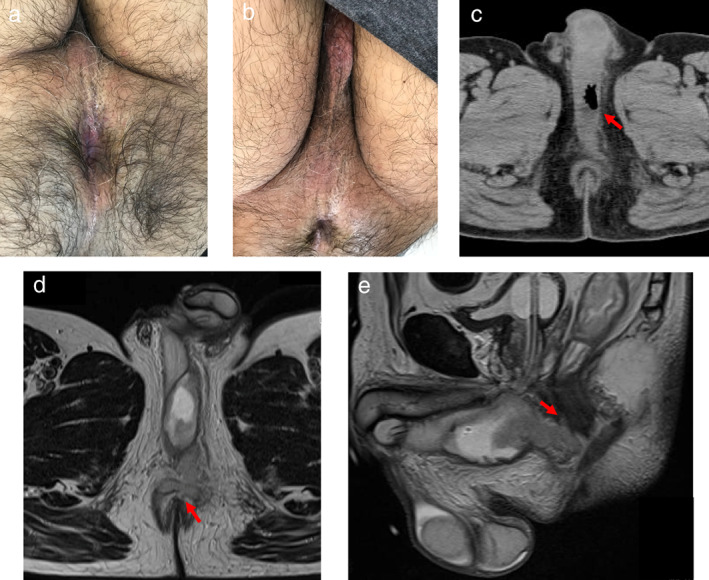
(a) No perianal redness is present, (b) but there is mild erythema of the perineum. (c) Computed tomography demonstrates a low‐density area with air in the perineum (red arrow). (d) Magnetic resonance imaging reveals continuity of the abscess with the anal canal at 12 o' clock (red arrow), (e) which is confirmed on the sagittal view (red arrow).
